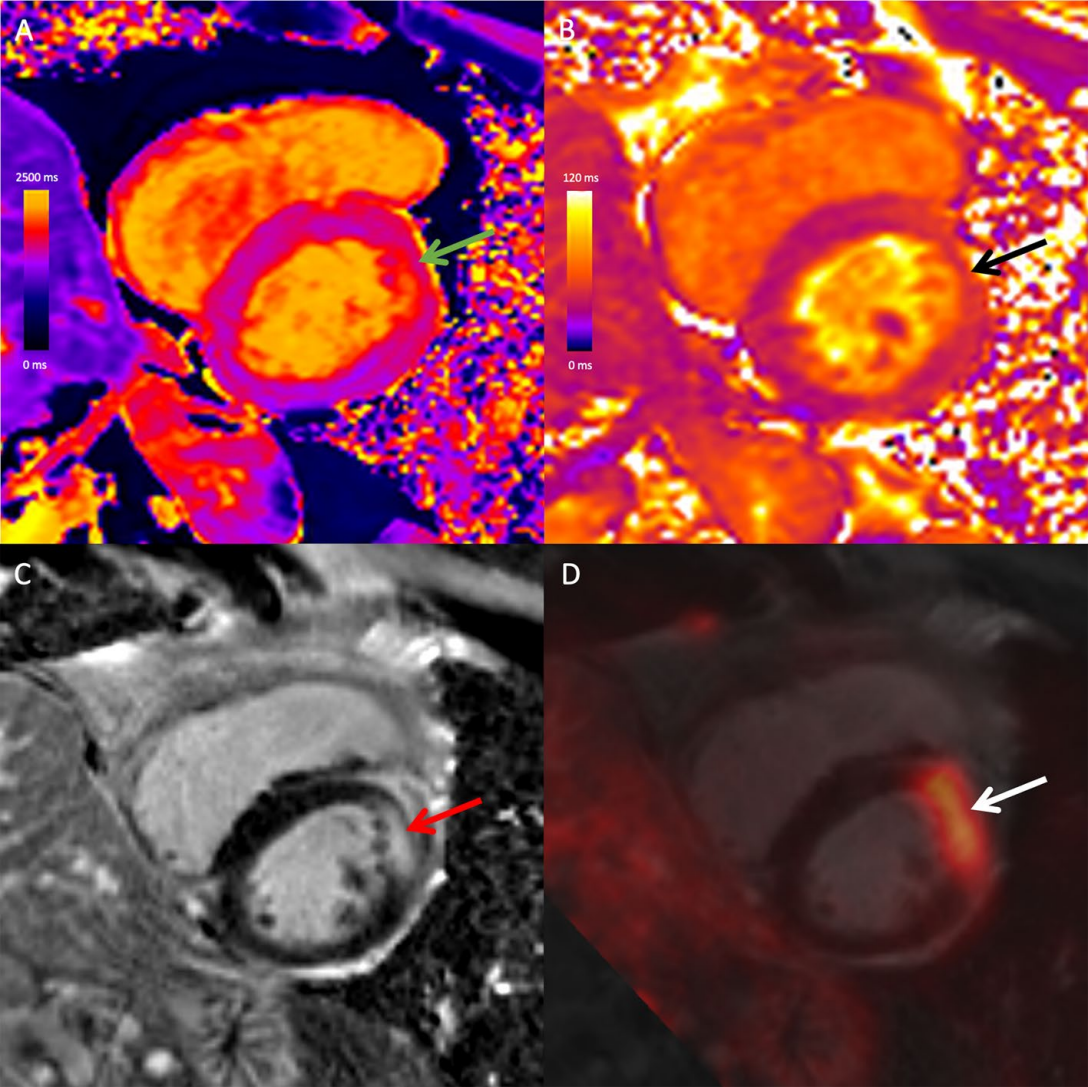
Combined 18F-FDG PET/MRI images in a 67-year-old male with cardiac and extra-cardiac sarcoidosis. Mid-ventricular short-axis native T1 map (A), native T2 map (B), late gadolinium-enhanced (LGE) image (C), and fused 18F-FDG PET and LGE image (D) demonstrate high T1 (green arrow), high T2 (black arrow), mid-wall LGE (red arrow) and co-localizing focal FDG uptake (white arrow) at the mid-anterior wall. There was extra-cardiac FDG uptake in mediastinal and hilar lymph nodes (not shown). The study was positive on both PET and MRI, likely reflecting active cardiac sarcoidosis with myocardial inflammation
